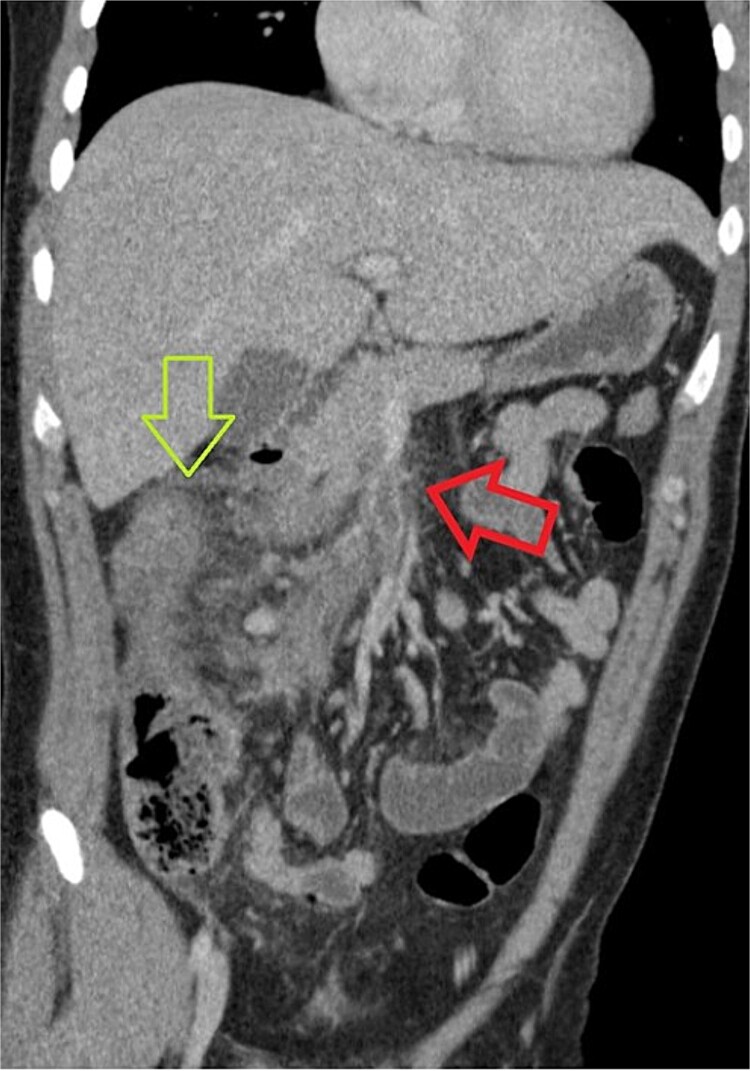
CT of abdomen and pelvis showing a thrombus within the superior mesenteric vein, identified with a red (or horizontal) arrow. Some linear foci which may be branching of hypoattenuation in segment 8 of the liver which possibly related to distal branches of the right portal vein and could represent early portal vein thrombosis. The green (or vertical) arrow identifies colonic edema at the hepatic flexure.
